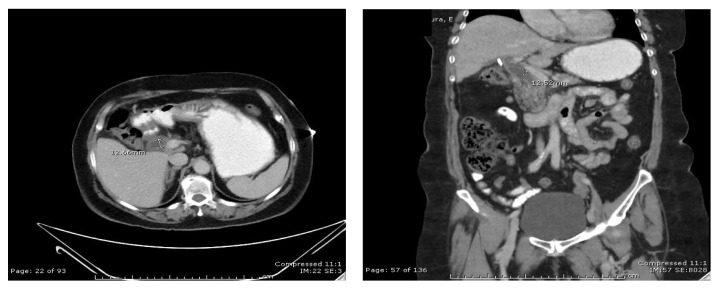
Postoperative CT scan abdomen with oral and IV contrast (axial and coronal views) showing dilated common hepatic duct (12.5 mm diameter).
